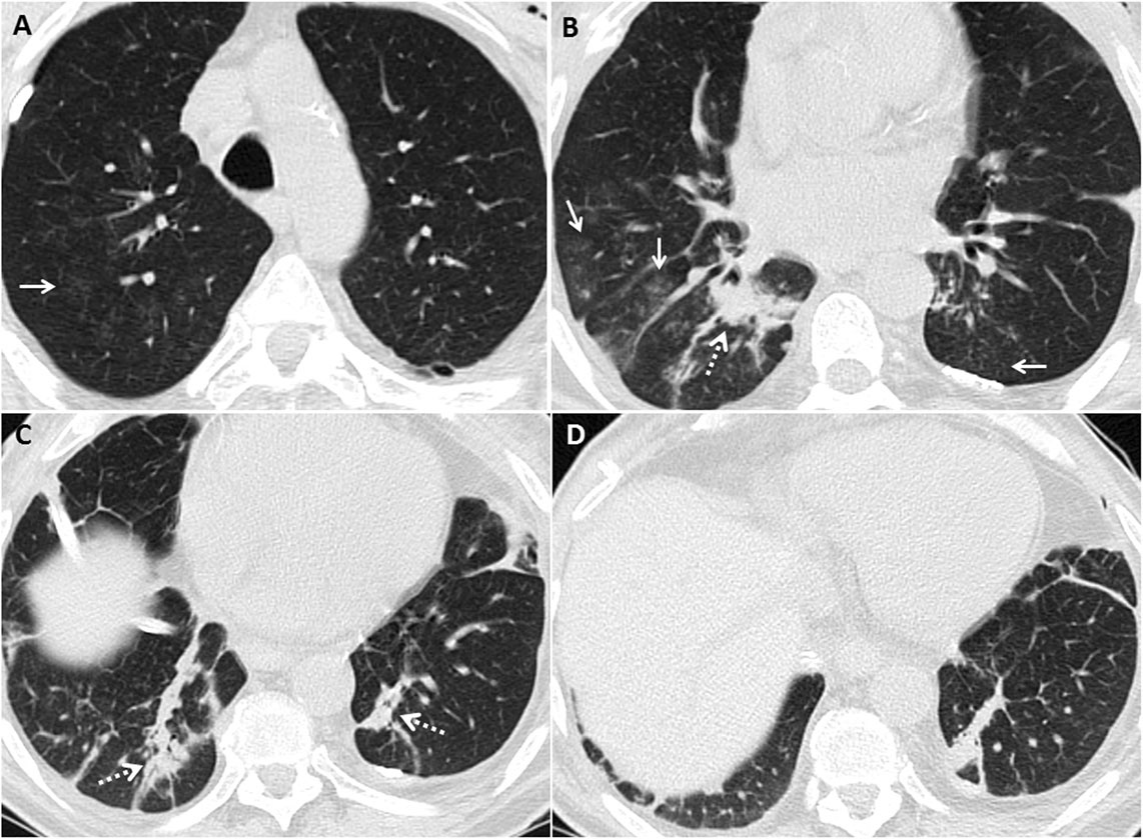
Patient with bilateral lung transplant and mild acute cellular rejection. Axial CT images (a–d) show asymmetric (right more than left) patchy and nodular peribronchial ground glass opacities (arrows) with basilar peribronchial consolidative opacities (arrowheads) and mild bibasilar septal thickening. Transbronchial biopsy of both lower lobes showed mild acute cellular rejection (grade A2 B0)
